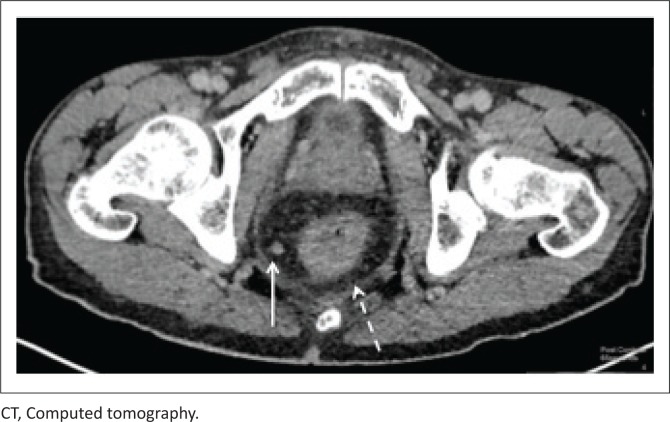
Axial reconstruction, portovenous-enhanced CT imaging of a 50-year-old male patient. The image demonstrates rectal thickening (dashed arrow) and an enhancing mesorectal node (solid arrow).
